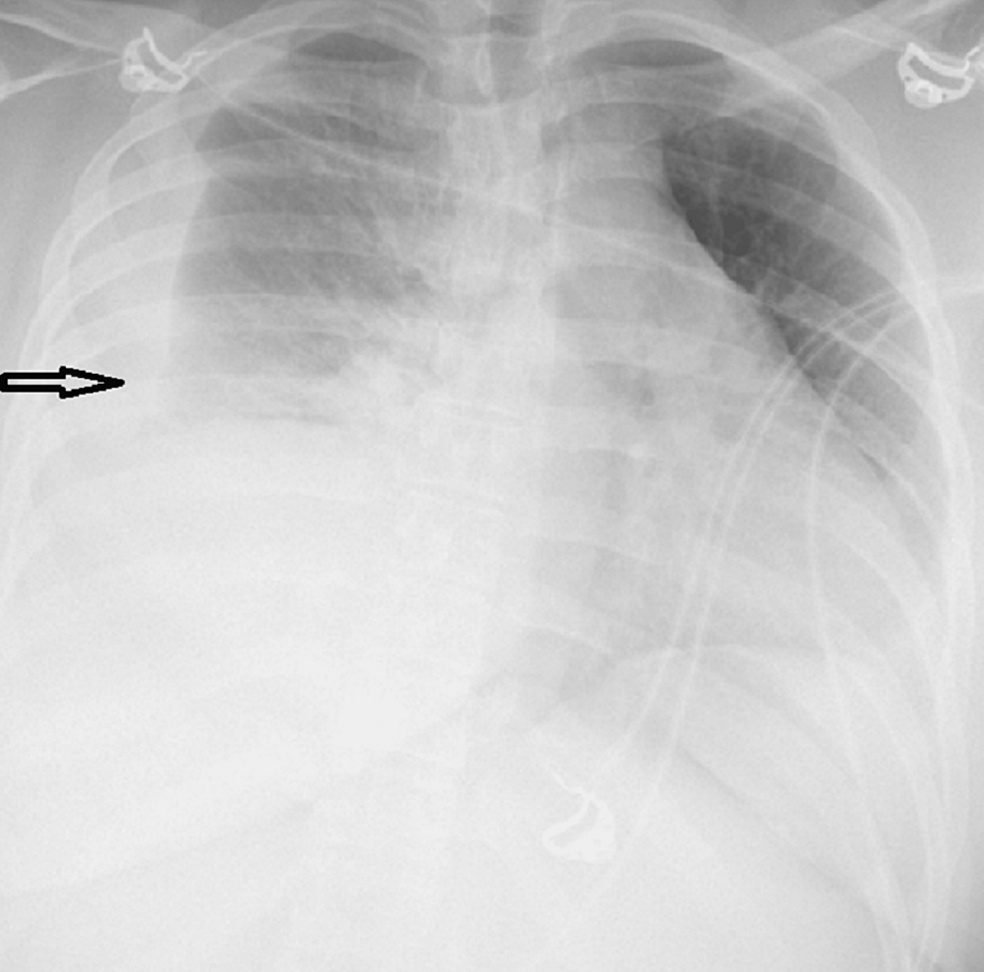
Chest x-ray done within 24 hours of initial presentation showing large right-sided pleural effusion (arrow)
